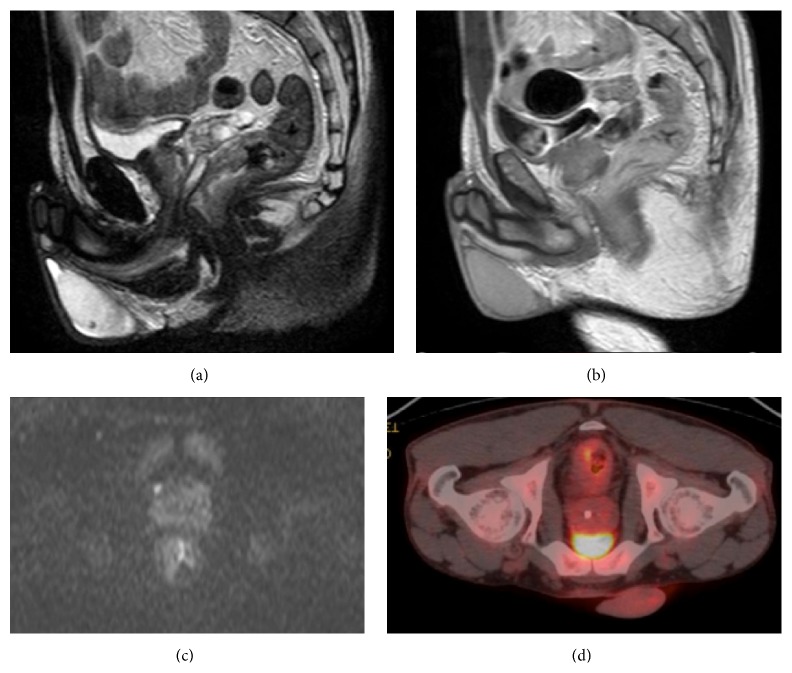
(a) T2 weighted sagittal plan with isointense rectal wall thickness; (b) T1 weighted sagittal plan after gadolinium administration with homogeneous contrast enhancement of the rectal walls; (c) Axial diffusion-weighted image (*b* = 1000) with no hyper intensity of the rectal wall; (d) 18F FDG PET-CT with focal FDG accumulation in the rectal wall.
